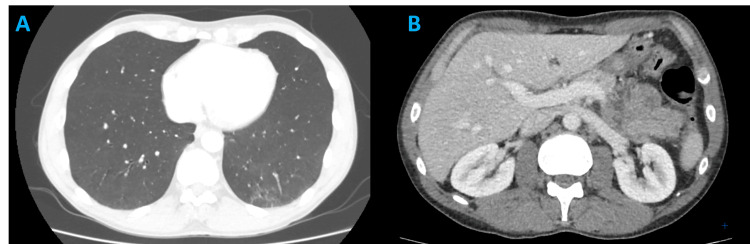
Contrast-enhanced CT scan. (A) Axial chest CT image in lung window showing no evidence of pulmonary nodules or metastatic lesions. (B) Axial abdominal CT image in portal venous phase demonstrating homogeneous liver parenchyma without focal lesions, consistent with the absence of hepatic metastases. CT: computed tomography
